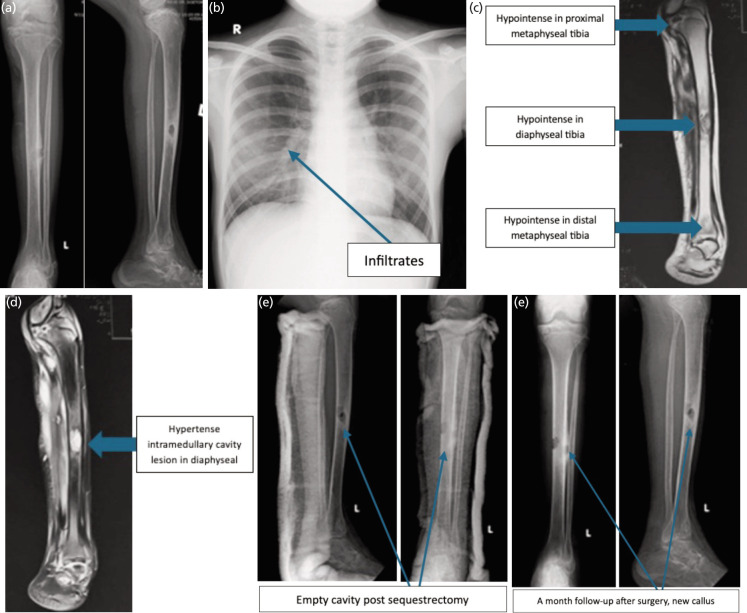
(a) Cruris, foot, and ankle before surgery, (b) Chest radiograph after 12 month of anti-TBC drug, (c) MRI T1-weighted image coronal view of tibia. The blue arrow shown hypointense in proximal and distal metaphyseal and diaphyseal of tibia. MRI T2 weighted image's coronal view of tibia. (d) The blue arrow showing hyperintense intramedullary cavity lesion. (e) empty cavity after sequestrectomy, (f) bone callus formed after a month of surgery.
